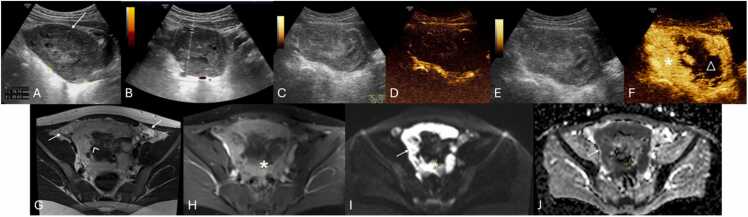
(A–J) Elective pelvic transabdominal ultrasound, CEUS, and pelvic MRI in a 9-year-old female presenting with pelvic pain for 15 days. She had previous a nephrectomy for Wilms tumor and was on follow-up. During ultrasound (A), a complex mass was noted in the pelvic floor (arrow in A), and the ovaries were not clearly visualized due to acoustic shadowing from adjacent bowels, raising concern for ovarian torsion with an associated adnexal mass. Due to MRI not being readily available, a CEUS was performed the same day (B–F), revealing a mass with intense peripheral enhancement (* in F) and no central enhancement (△ in F), likely due to necrotic components. The diagnosis of a pelvic tumor nature was placed. (G–J) Axial T2-weighted (G), contrast-enhanced T1-weighted FS and diffusion-weighted with respective ADC map (I and J) MR images obtained the following day are shown, confirming the presence of an independent pelvic mass (arrowhead in G) adjacent to the ovaries (arrow in G) with peripheral enhancement (* in H) and diffusion restriction (arrow in I and J). Surgery confirmed a 10 cm pelvic Wilms recurrence, with extensive necrosis, capsular rupture, and peritoneal implants.
